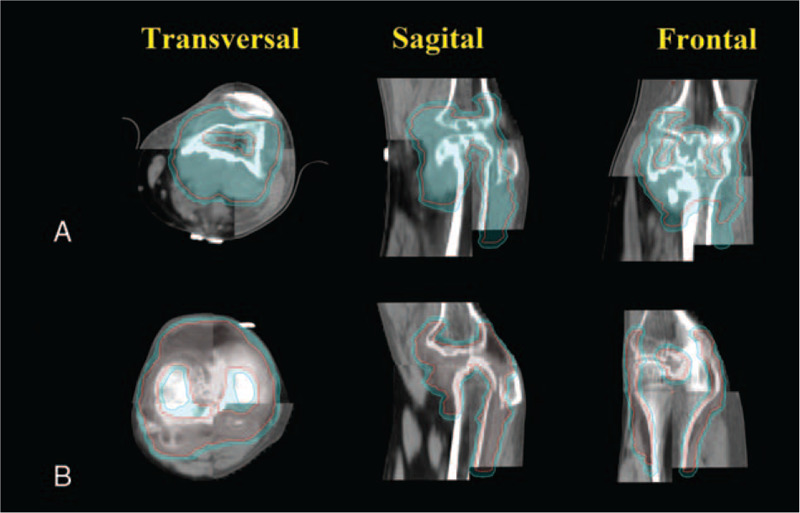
Image-guided radiotherapy (IGRT) could reduce errors caused by setup processes: before radiotherapy, the cone-beam CT (CBCT) images were used to register with the IMRT treatment plan images, and the position error was reduced to less than 1 mm.
